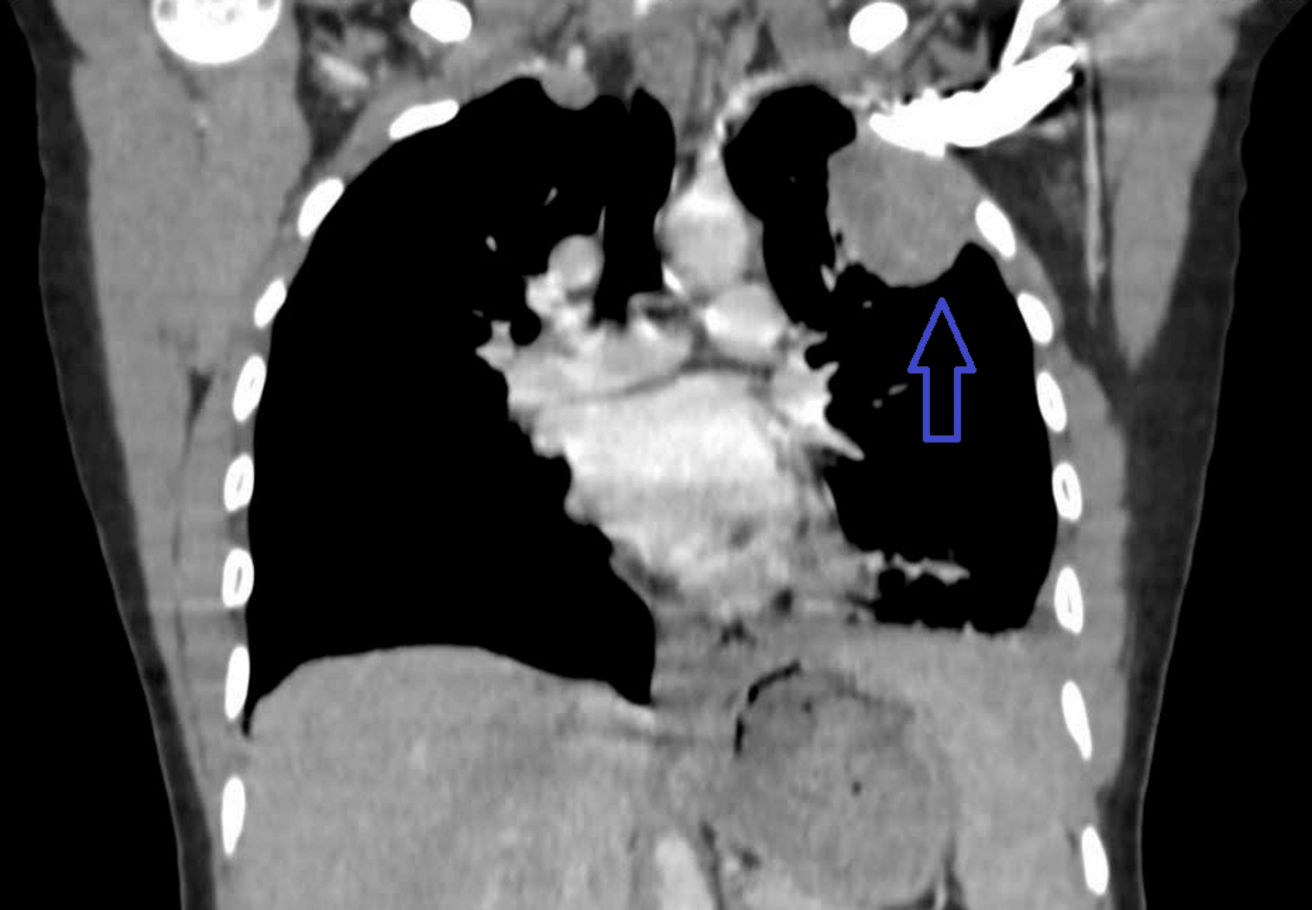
CT scan showing a mass in the left upper lobe CT = Computed tomography
